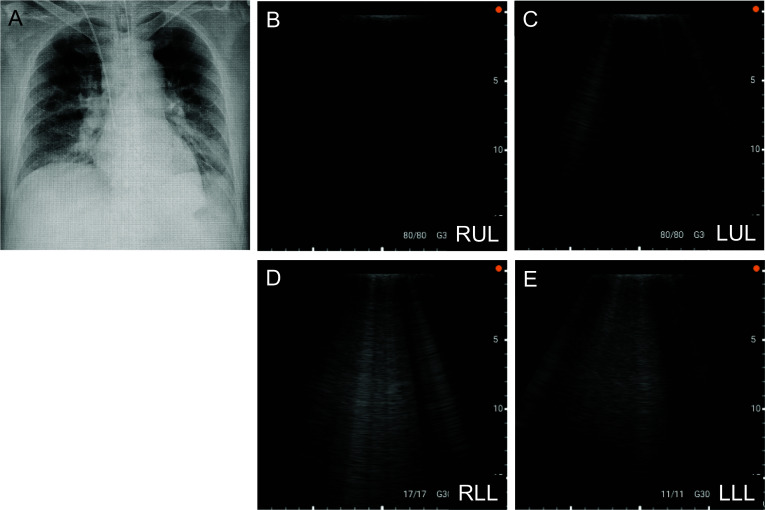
Chest radiographs showing increased infiltrative shadows on the mediastinal side from the right lung hilum to the middle and lower lung fields and the left middle and lower lung fields (A). Lung ultrasound showing that RUL (B) and LUL (C) were in the hypoechoic area. In contrast, RLL (D) and LLL (E) show numerous B-lines, indicating thickening of the interlobular septal wall and fluid retention in the alveoli. RUL: right upper lobe, RLL: right lower lobe, LUL: left upper lobe, LLL: left lower lobe
